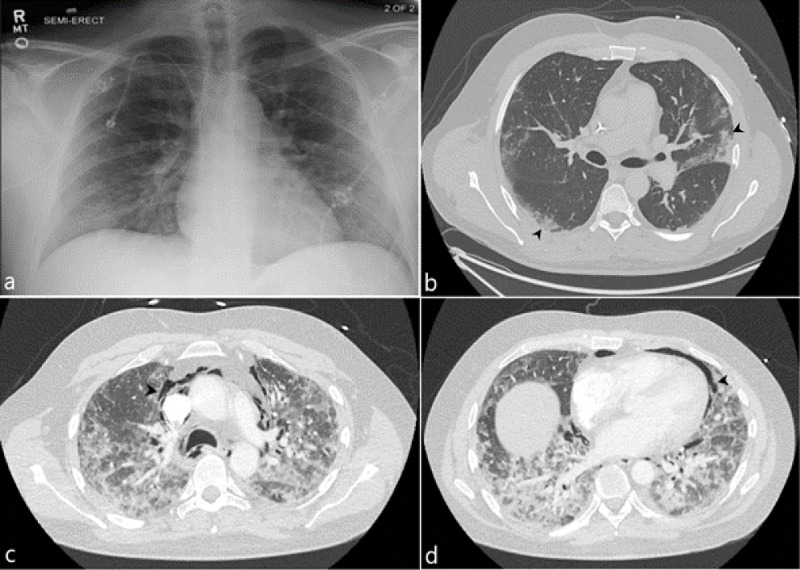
37-year-old male with spontaneous pneumomediastinum (a) Initial admission frontal chest radiograph demonstrates no significant cardiopulmonary findings. (b) Initial axial CT image of the chest demonstrates peripheral ground glass opacities (black arrowhead). (c,d) Follow up axial CT images of the chest demonstrate lucencies within the mediastinum corresponding to a new spontaneous pneumomediastinum (representative arrowheads).
